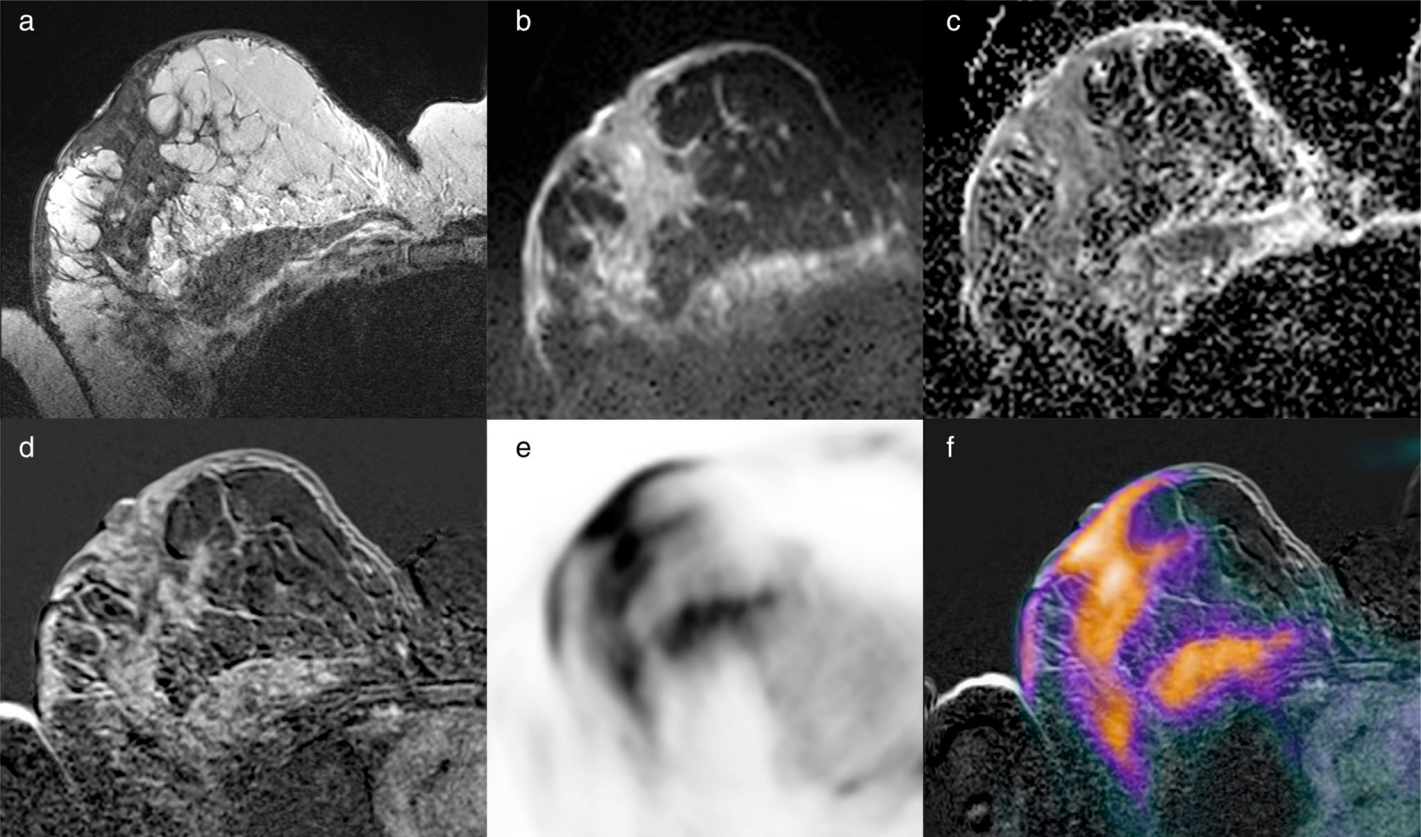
An example of multiparametric imaging obtained using hybrid ^18^F‐FDG PET/MRI. A 56‐year‐old woman with invasive ductal breast cancer (G3, triple negative) in the right breast associated with pectoral muscle infiltration and skin thickening, shown on axial (a) fat‐saturated T2‐weighted turbo spin‐echo imaging, (b) diffusion‐weighted echo‐planar imaging and (c) apparent diffusion coefficient mapping, (d) contrast‐enhanced T1‐weighted imaging, (e) PET imaging, and (f) fused PET/MRI imaging.
